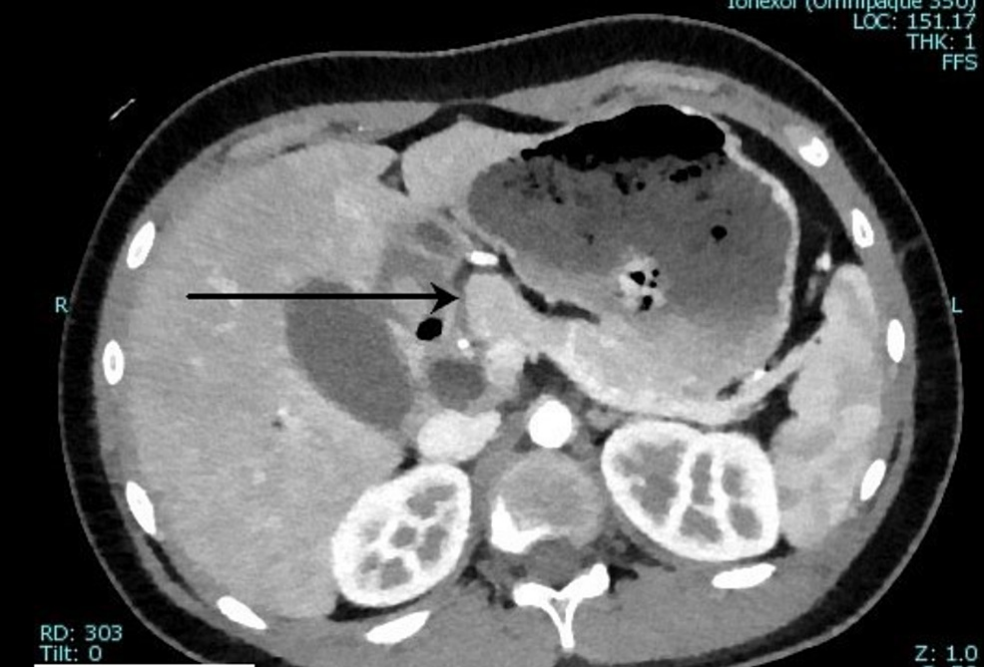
Pancreatic head mass on CT. Arrow shows a pancreatic head mass measuring 2.8 × 2 × 3 cm. The mass is ill-defined and mildly hypoenhancing on the arterial phase. CT: computed tomography.
